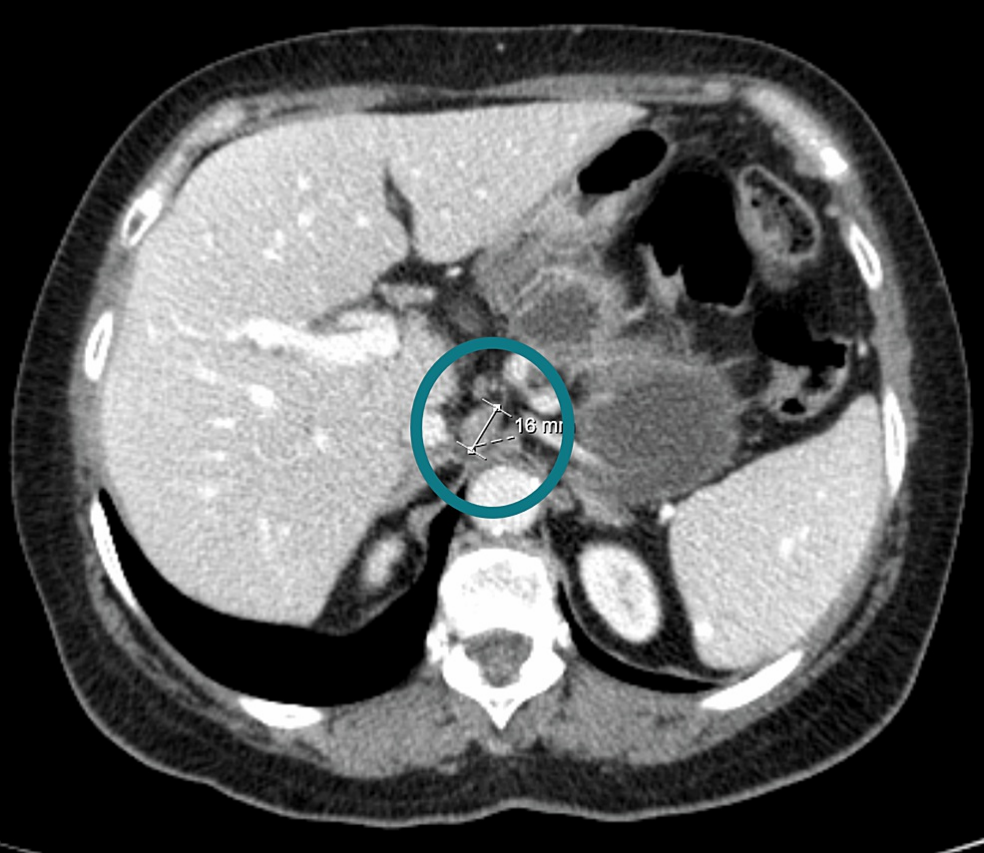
Abdominal CT from 2018, before the diagnosis, where intercavalportal adenopathies were identified. (Circle)
